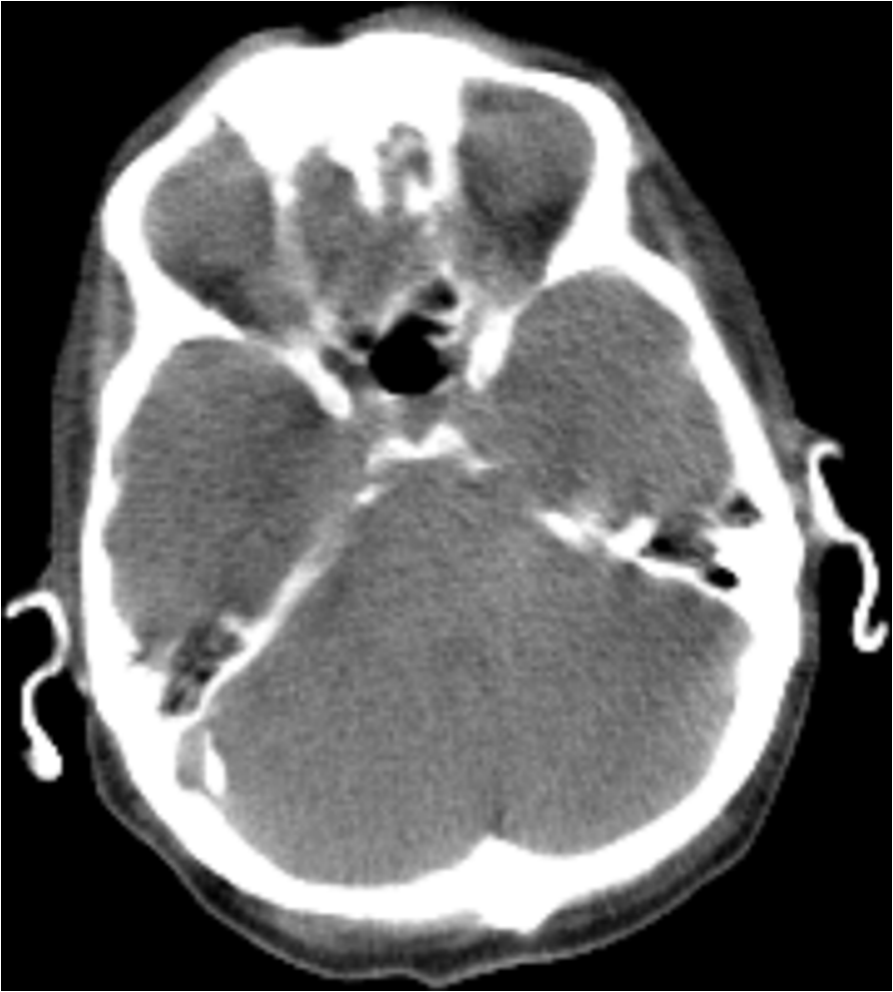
Computed tomography scan of the cerebrum displayed fully calcified auricles.
